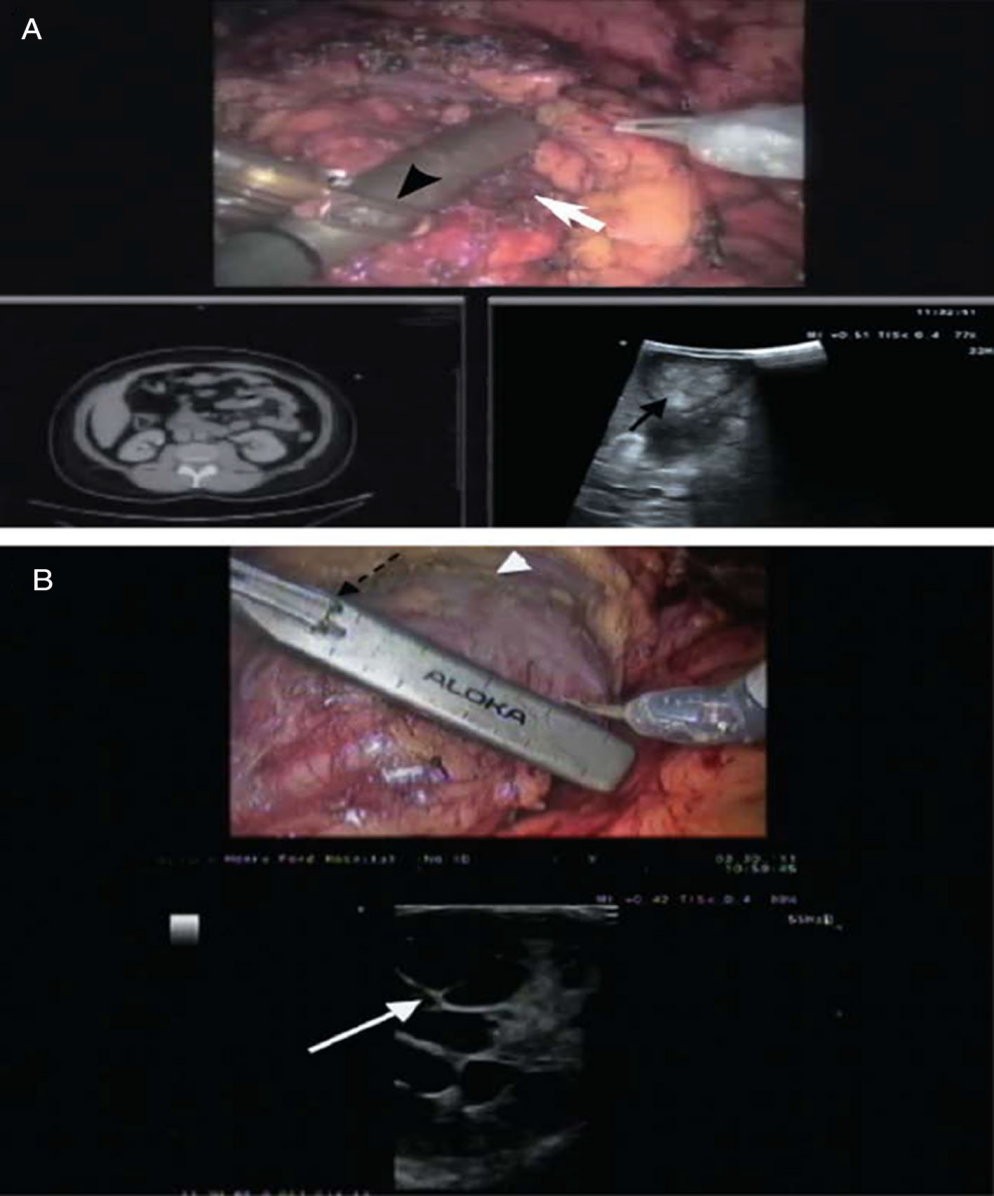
a Laparoscopic ultrasound probe being used for a right renal mass identification (a solid *white arrow* on the upper console image; solid *black arrow* on lower TilePro ultrasound image). The surgeon is trying to grab the laparoscopic probe with the robotic instrument to adjust the position (*black arrow-head*). b Robotic ultrasound probe being used to identify a right renal cystic renal cell carcinoma (*solid white arrow*). The robotic instrument is engaged with the notch on the probe (*dashed black arrow*), allowing the surgeon to independently maneuver the probe to identify tumor margins. The *arrow head* notes the scored resection margin of the far side of the tumor [8]
